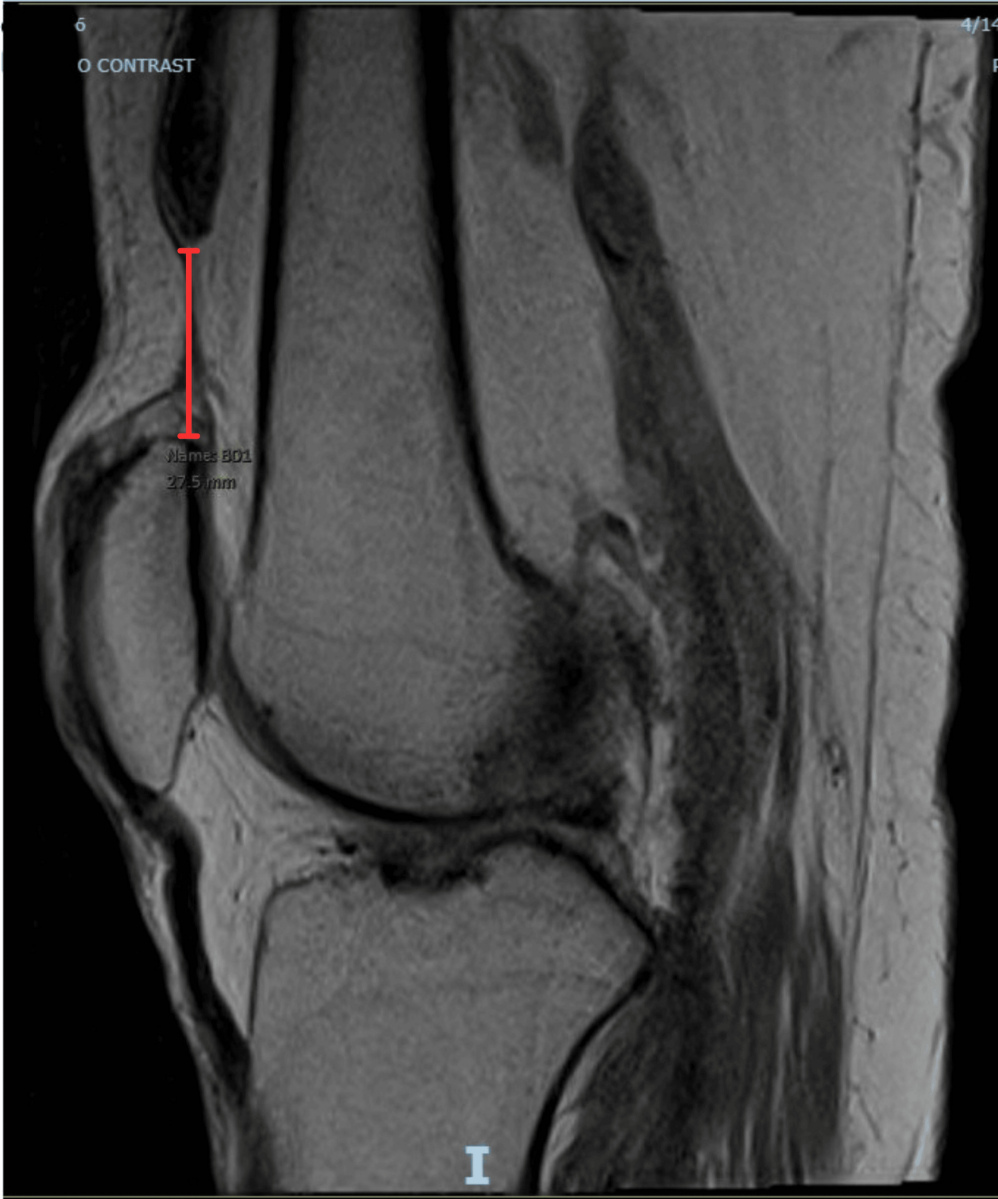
Right Knee MRI, Lateral View with Measurement Lateral view of right knee MRI (T1) again, with extent of retraction as measured by reading radiologist (red line, measuring 2.75 cm).
